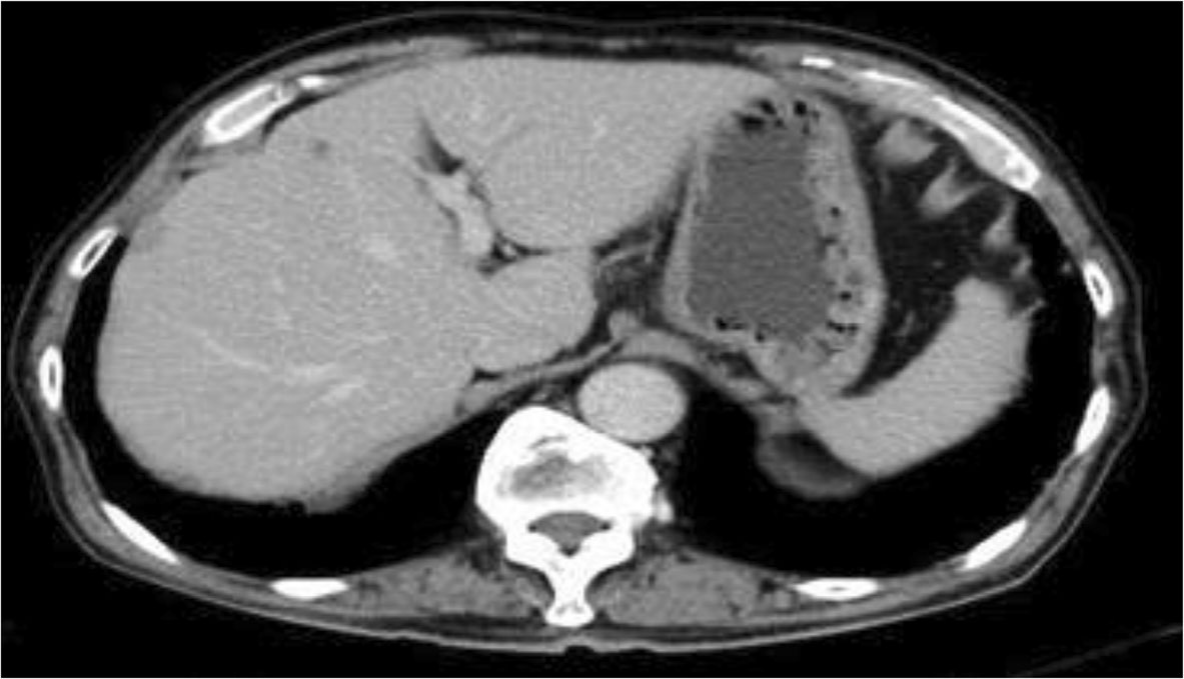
Contrast-enhanced computed tomography image of the abdomen 3 months after the initial visit showing regression of tumors
